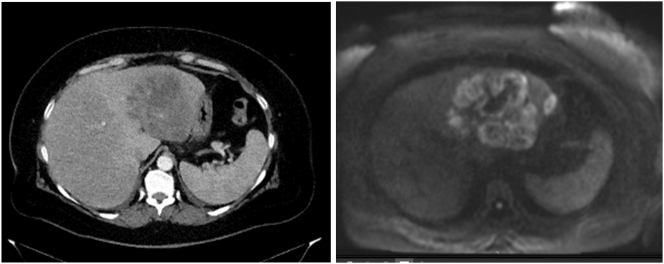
CT scan upon admission to the hospital (left) and MRI (right) showing a polylobulated, cystic, partly solid tumourous process of the left lobe of the liver.
